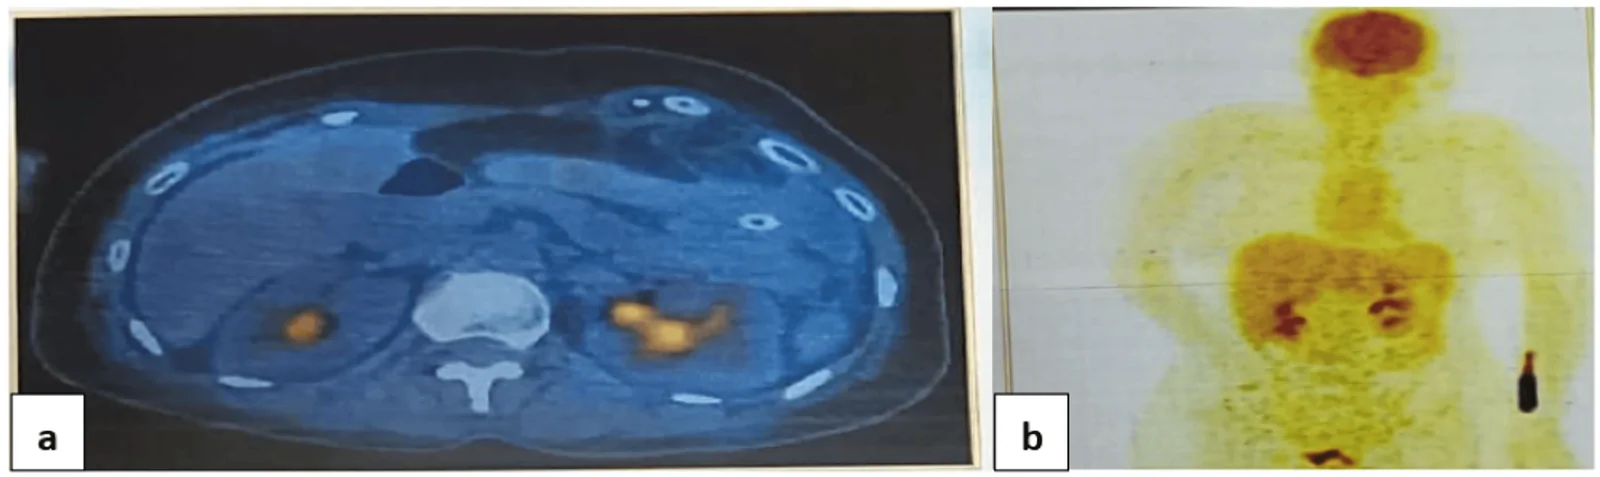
PET-CT scan taken for assessing metastasis The figure shows the PET-CT scan taken for the patient. Image a shows an abdominal section with no significant FDG avidity in the liver, spleen, pancreas, or other organs. Image b shows a full body scan including the brain, where there was no evidence of increased FDG avidity or metabolically active lesions anywhere in the body. PET: positron emission tomography; CT: computed tomography
